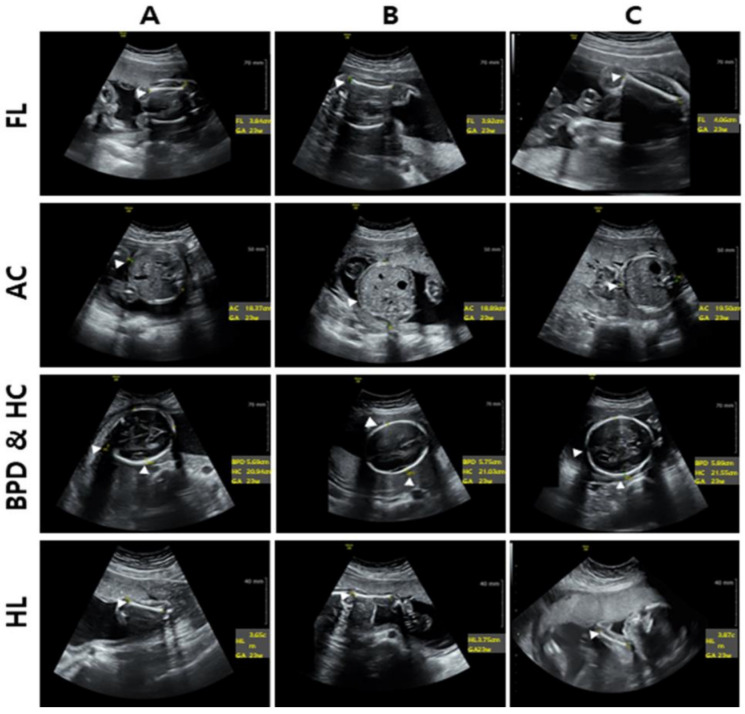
The correlation between vitamin D status and precision of ultrasound imaging in 23-week pregnant women. (A) deficient, (B) insufficient, (C) sufficient were defined as 25(OH)D_3_ concentration <20, 21–30, and >30 ng/mL, respectively. FL, Femur length; BPD, Biparietal diameter; HC, Head circumference; AC, Abdominal circumference; HL, Humerus length.
